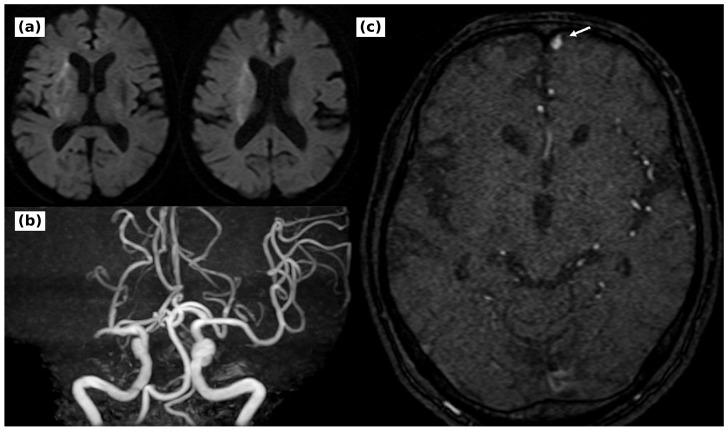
Initial magnetic resonance imaging and retrospective review of time-of-flight magnetic resonance angiography. (a) Diffusion-weighted imaging showed faint hyperintensity in the right basal ganglia and corona radiata; the diffusion-weighted imaging-Alberta Stroke Program Early Computed Tomography Score was 9. (b) Magnetic resonance angiography suggested occlusion of the right M1 segment. (c) Retrospective review of the pretreatment time-of-flight source image demonstrated an abnormal vessel in the left frontal region (white arrow).
